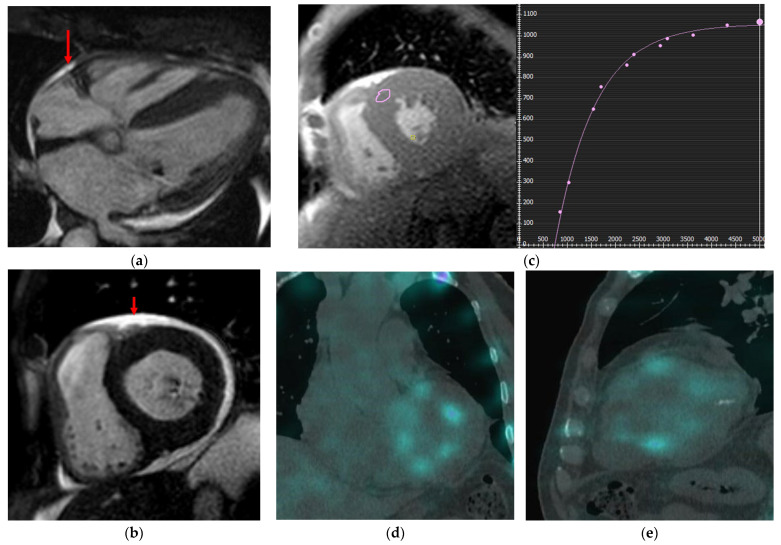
Cardiac amyloidosis. LGE sequences were not acquired due to impaired renal function. (a,b) Four-chamber (a) and short-axis (b) cine sequences: diffuse LV wall hypertrophy with left atrial dilatation, and mild pericardial effusion (arrow); (c) Native T1 mapping: elevated myocardial T1 values, reaching 1200 ms in the interventricular septum, suggesting amyloid infiltration and diffuse fibrotic changes; (d,e) coronal (d) and sagittal (e) reconstructions from Tc_m_^99^-DPD-SPECT: mild and patchy deposit of tracer in myocardium, compatible with amyloid infiltration.
